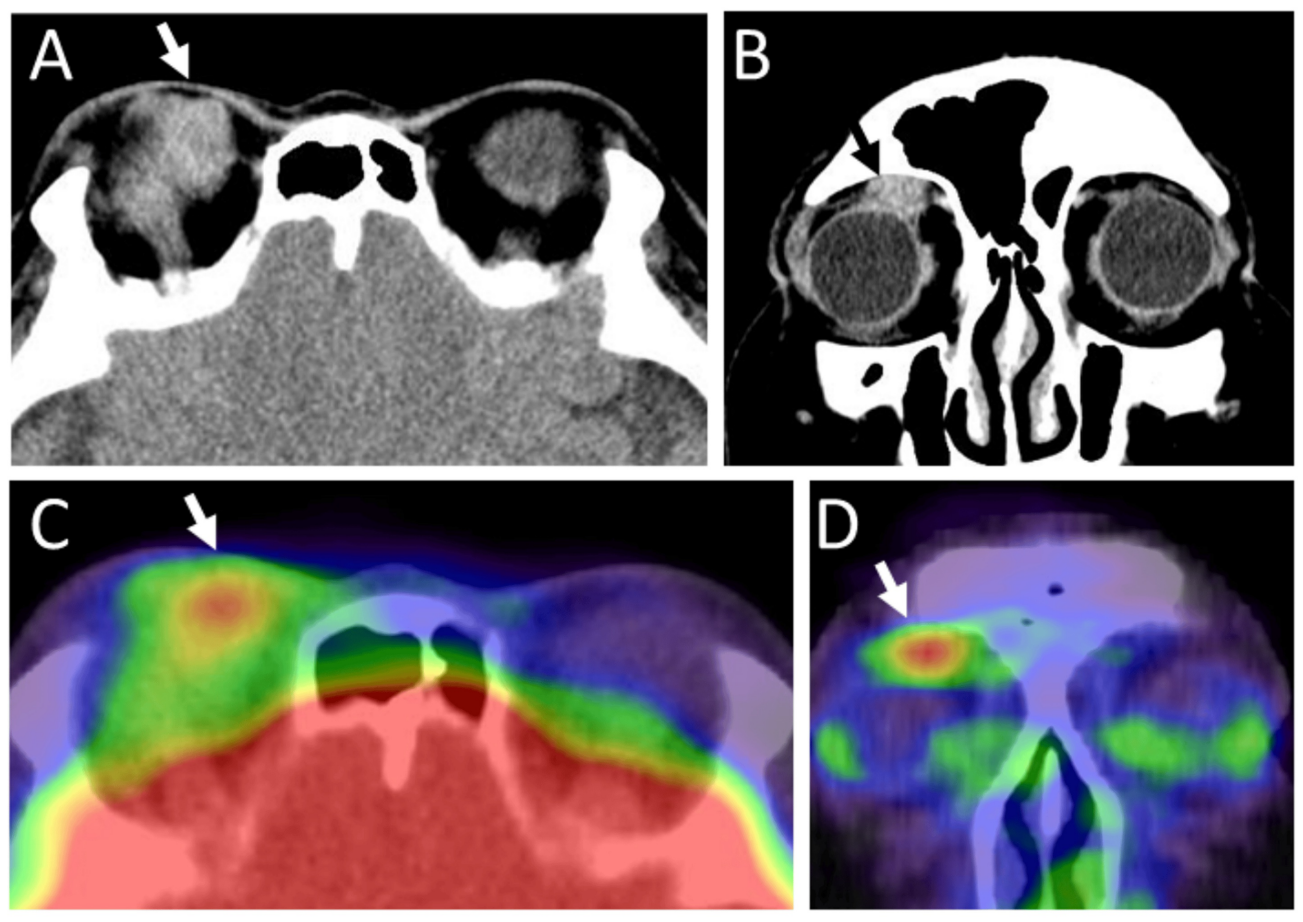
Computed tomography scans and positron emission tomography at age 51 Computed tomography scans in axial image (A) and coronal image (B) at age 51, showing an irregular-shaped mass (arrows) in the superior nasal orbit above the eyeball. Fluorodeoxyglucose positron emission tomography in axial image (C) and coronal image (D), showing only an abnormal uptake site (arrows) in the superior nasal orbit.
